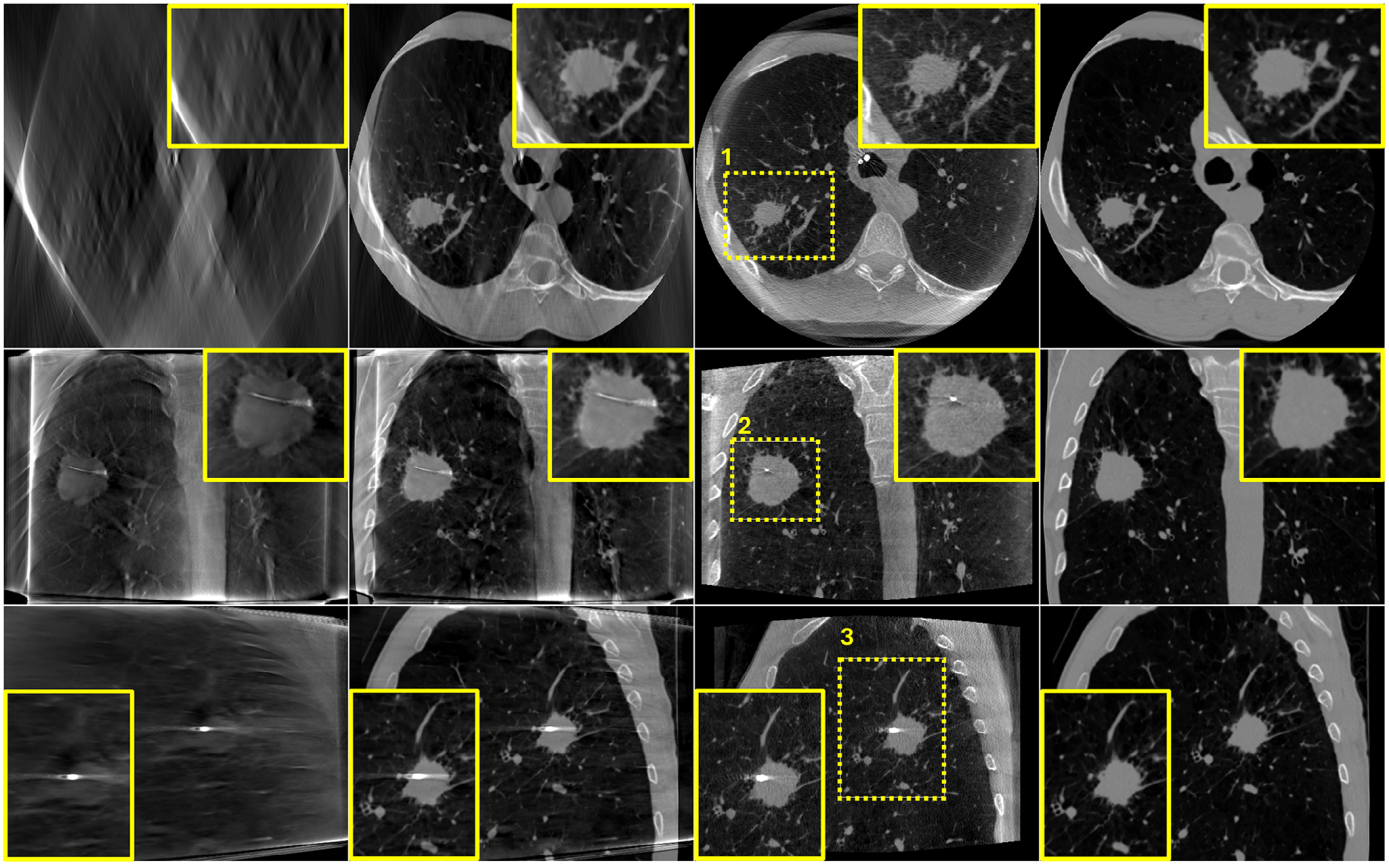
Patient bronchoscopy data reconstruction results using PL DTS data. (a) zero‐initialized ART, (b) prior‐aided reconstruction, (c) intraoperative CBCT, and (d) registered prior CT. Upper row: one axial slice. Middle row: one coronal slice. Lower row: one sagittal slice. Same slices are shown in (a), (b), (c), and (d). The display window range is [−1000 HU, 1000 HU]. ART, algebraic reconstruction technique; CBCT, cone‐beam computed tomography; CT, computed tomography; DTS, digital tomosynthesis; PL, pseudo‐linear.
